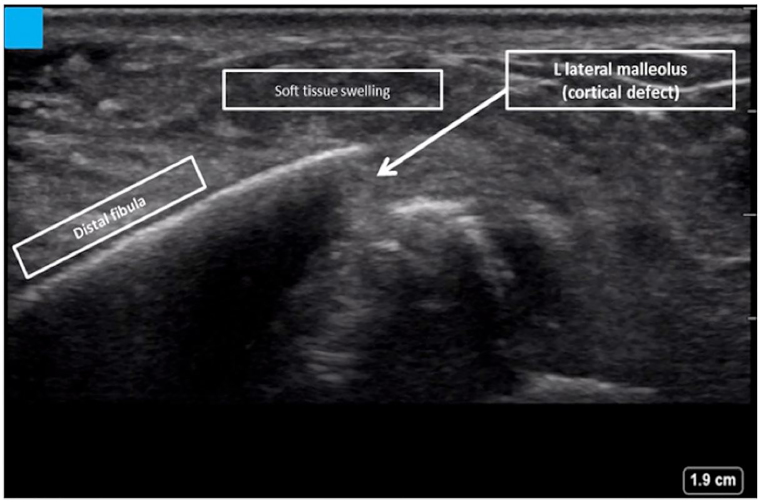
Longitudinal point-of-care ultrasound image of the distal fibula demonstrating a cortical defect.
